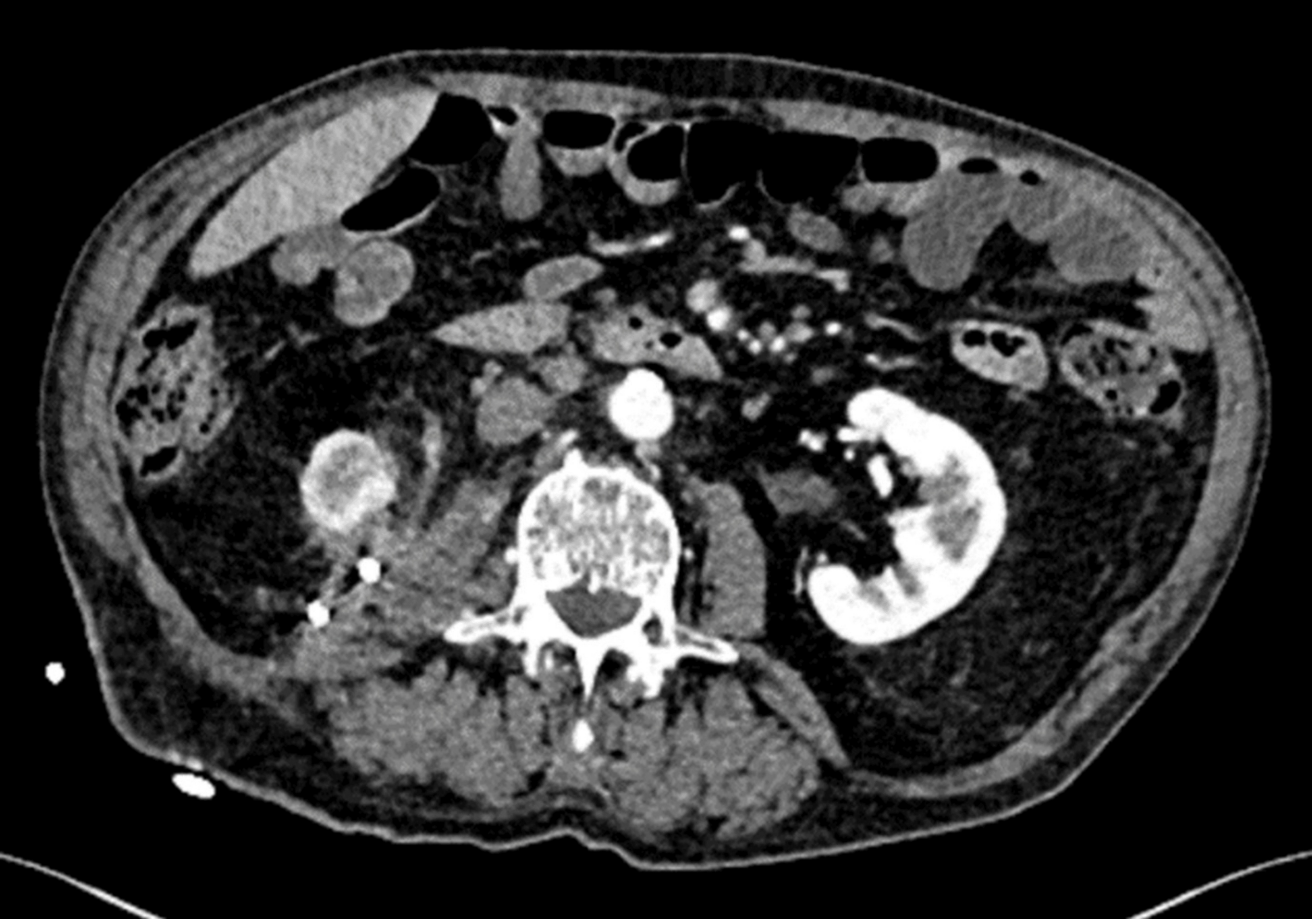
CT abdomen (axial view) in the portal venous phase CT demonstrates near complete resolution of the previous right retroperitoneal collection with trace residual perinephric fluid and stranding. The right-sided perinephric drain is visualised with the pigtail situated just posterior to the lower renal pole. CT, computed tomography
